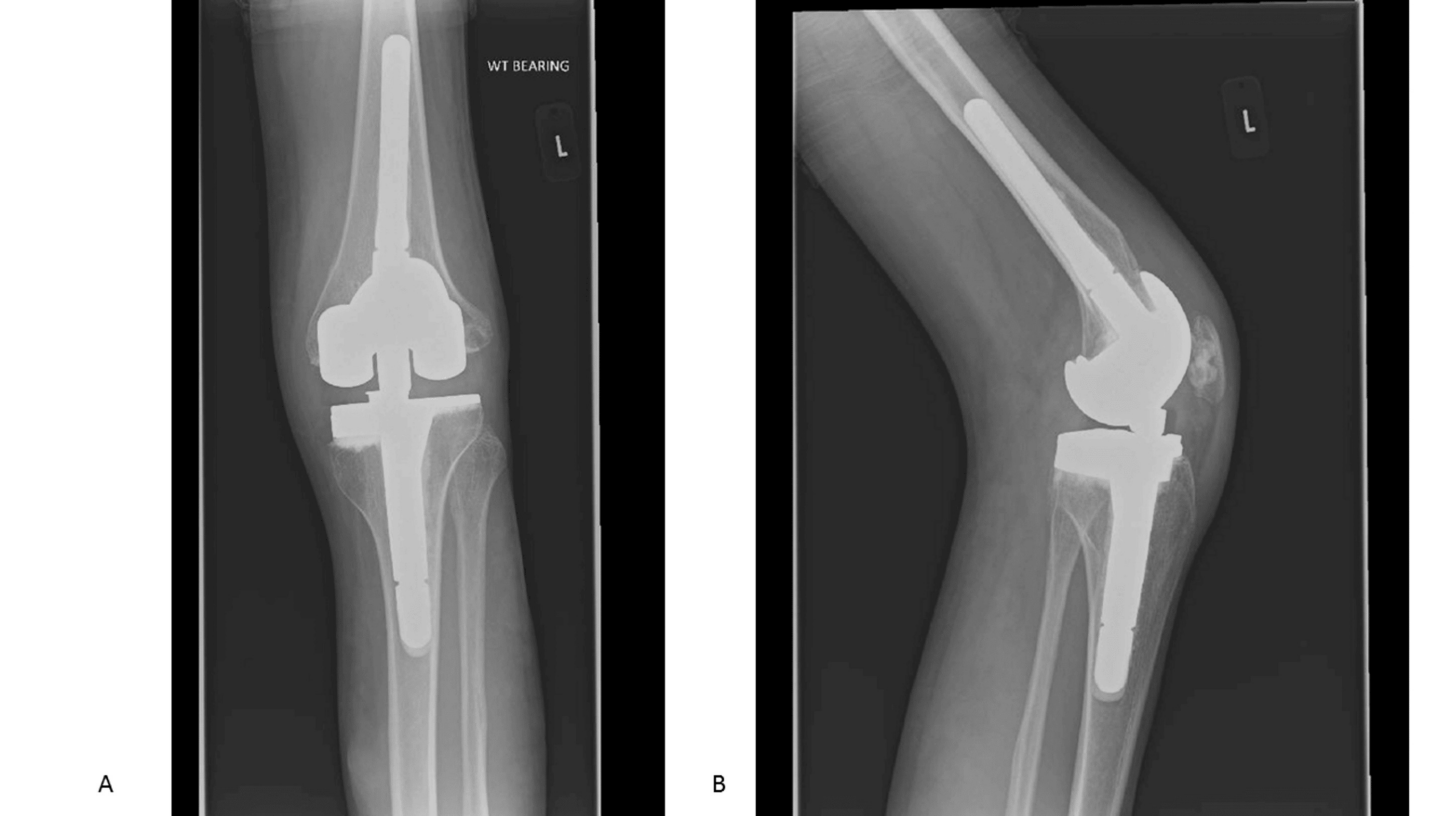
(A) Immediate postoperative anteroposterior X-ray of the left knee. (B) Immediate postoperative lateral X-ray of the left knee.
